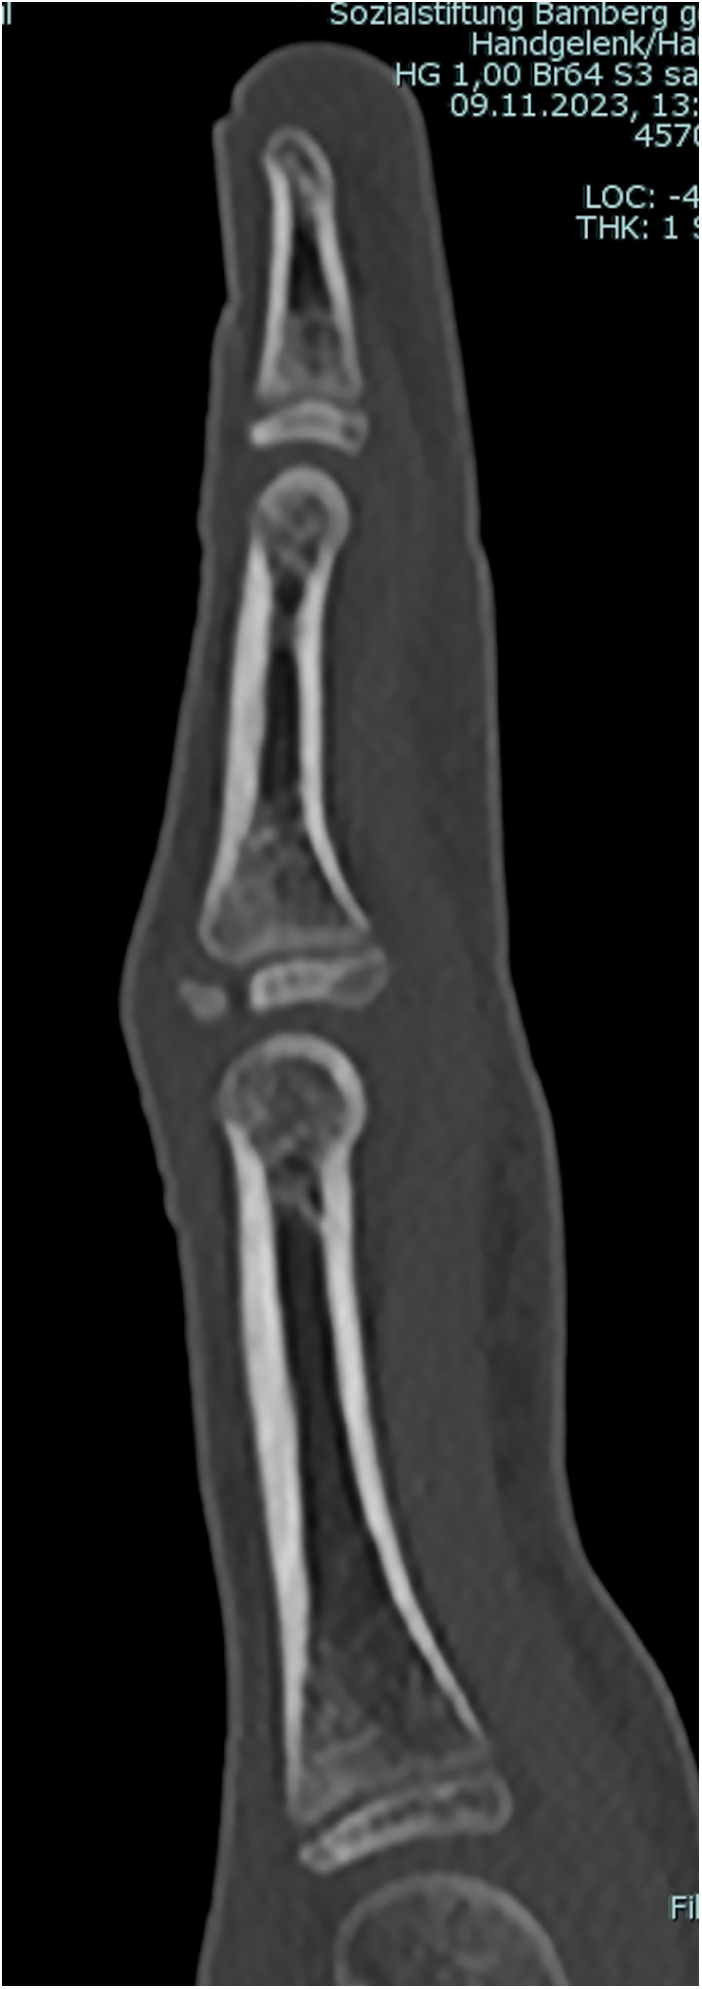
Radiographic sign 3. Displaced dorsal fracture of the epiphyseal-metaphyseal-complex (EPM) of the middle phalanx base (15 y old boy, right middle finger).
